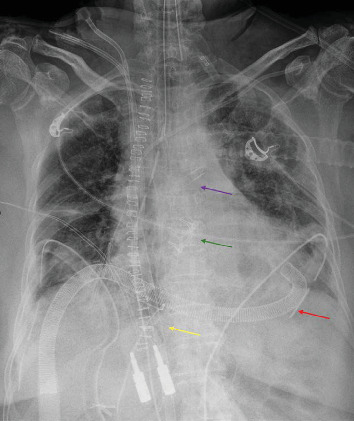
Chest X-ray 2 days after BiVAD placement showing partial improvement of pulmonary edema. Red arrow: inflow cannula of the LVAD (from the apex to the pump); yellow arrow: inflow cannula of the RVAD (from the inferior vena cava to the pump); purple arrow: outflow cannula of the RVAD (from the pump to the pulmonary artery); green arrow: bioprosthetic valve.
